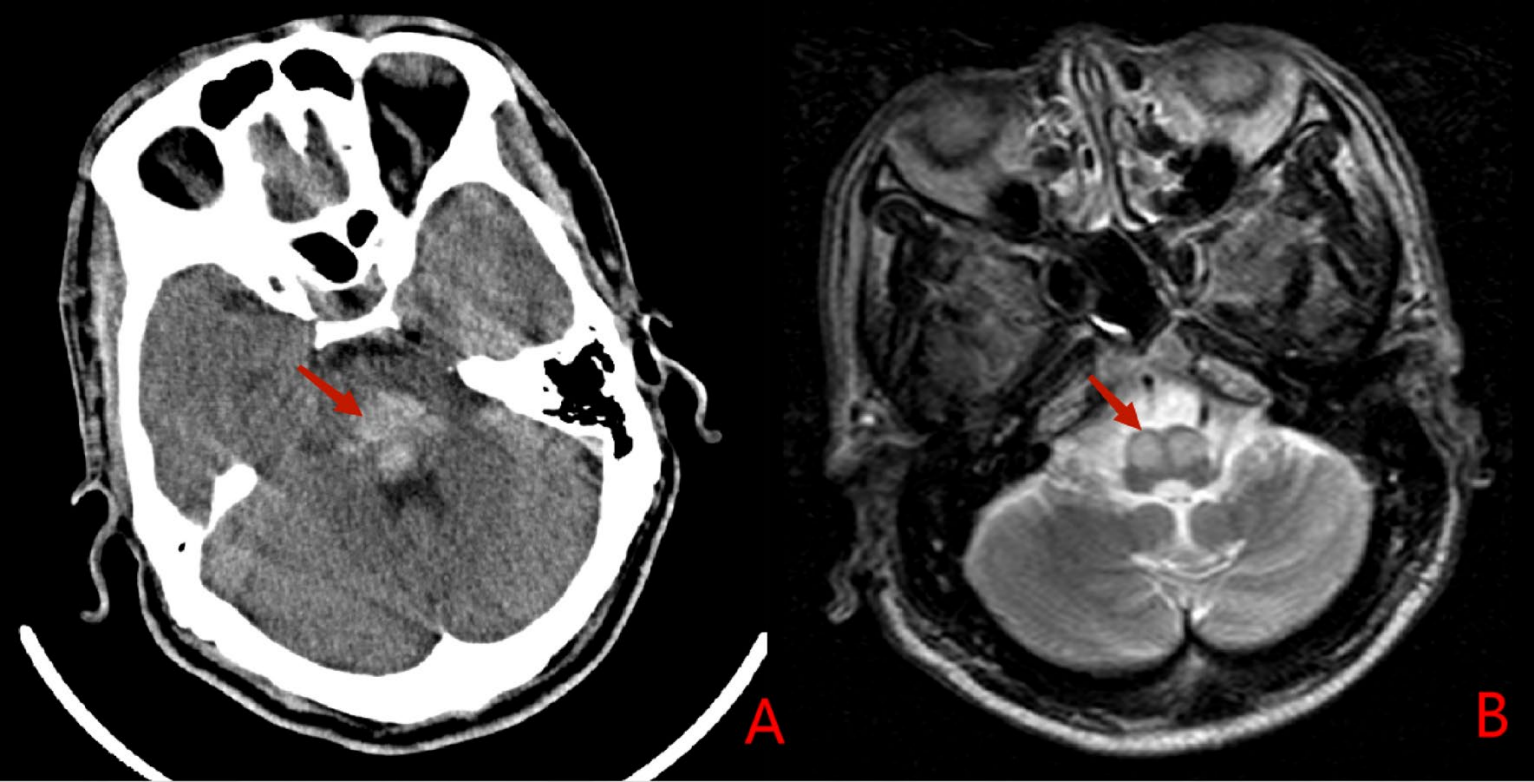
Case No.10, Head computed tomography (A) revealed pontine hemorrhage on both sides; T2WI (B) revealed hyperintensity on the both ION 7 months after the insult.
